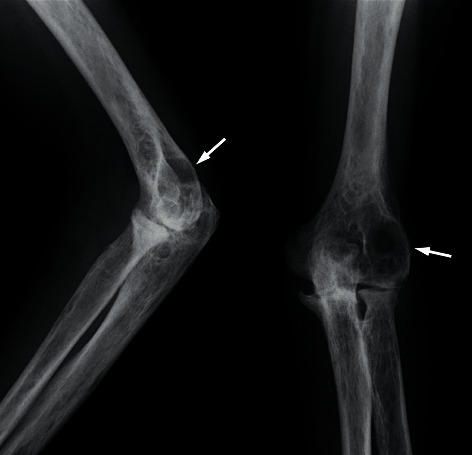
Plain radiograph of the left elbow at 10 months since the start of oral methotrexate. The large transparent area still remained, and poor repair is noted in the posterior lateral humeral bone (arrow).
